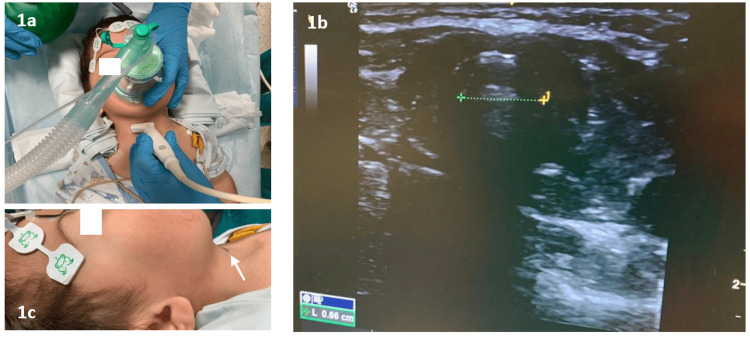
(a) Airway ultrasound after inhalation anesthesia with Sevoflurane, using a “hockey stick” probe (23 mm) to measure the tracheal diameter. (b) Ultrasound image of tracheal diameter measuring. (c) Arrow indicating cricotiroid membrane ultrasound-guided mark in the neck.
